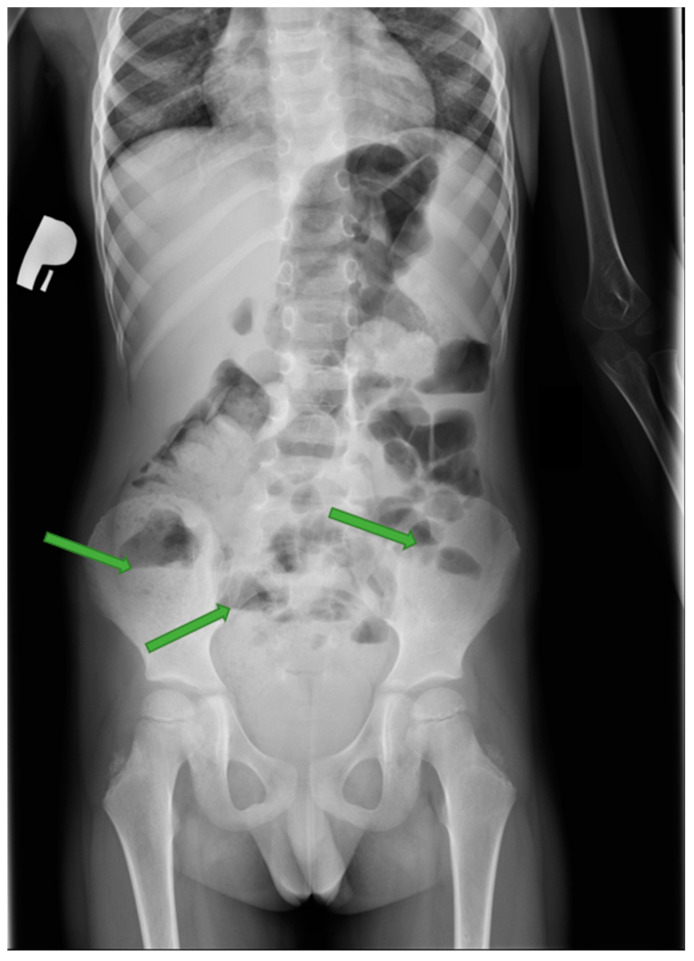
X-ray of the abdomen. Visible multiple levels of fluid in the intestinal loops (green arrows).
